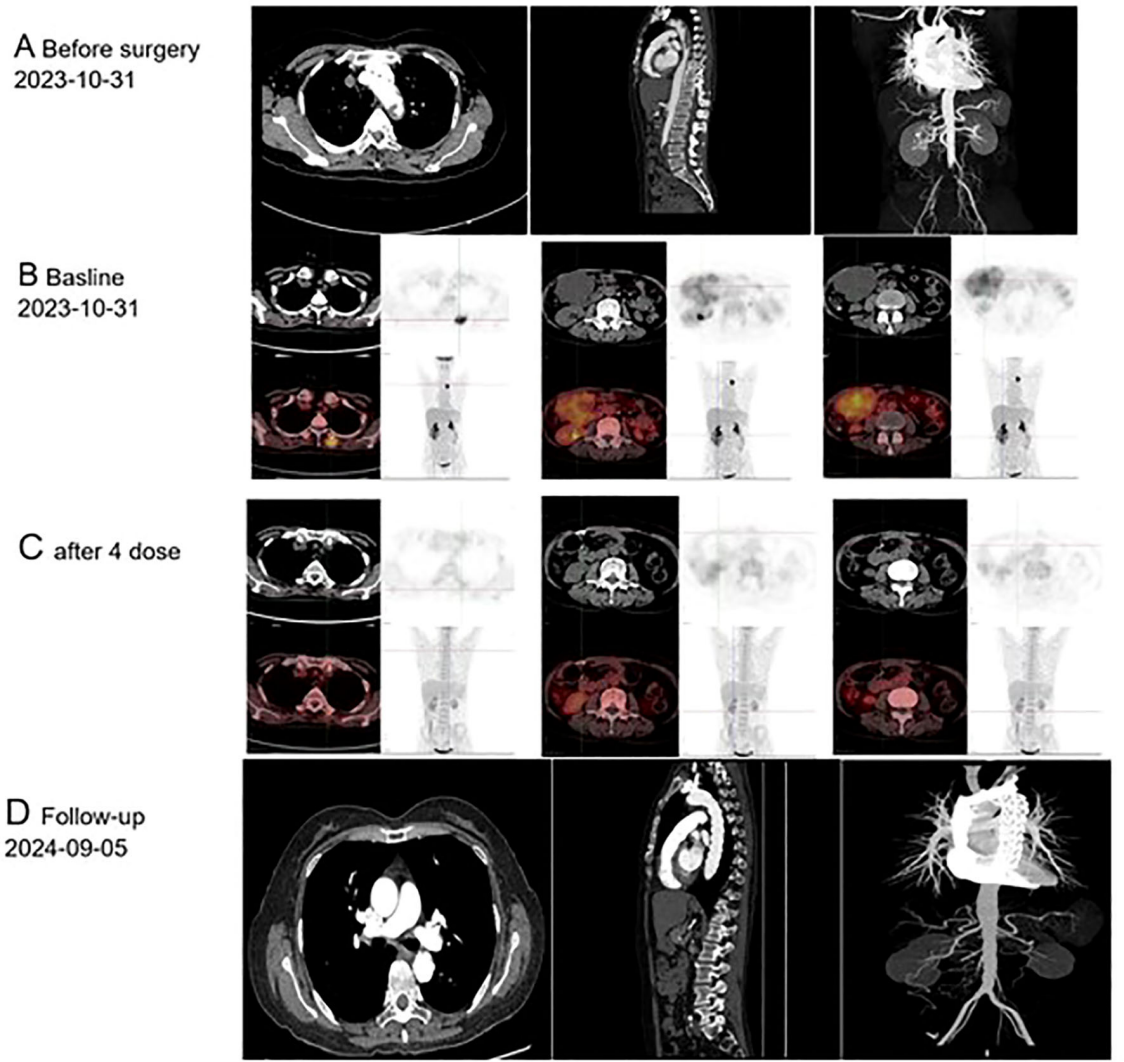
Imaging findings of primary endovascular sarcoma: (A) Computed Tomography conducted prior to October 31, 2023; (B) Positron Emission Tomography performed before December 14, 2023; (C) Positron Emission Tomography following four cycles of postoperative chemotherapy; (D) Follow-up care, September 5th, 2024.
